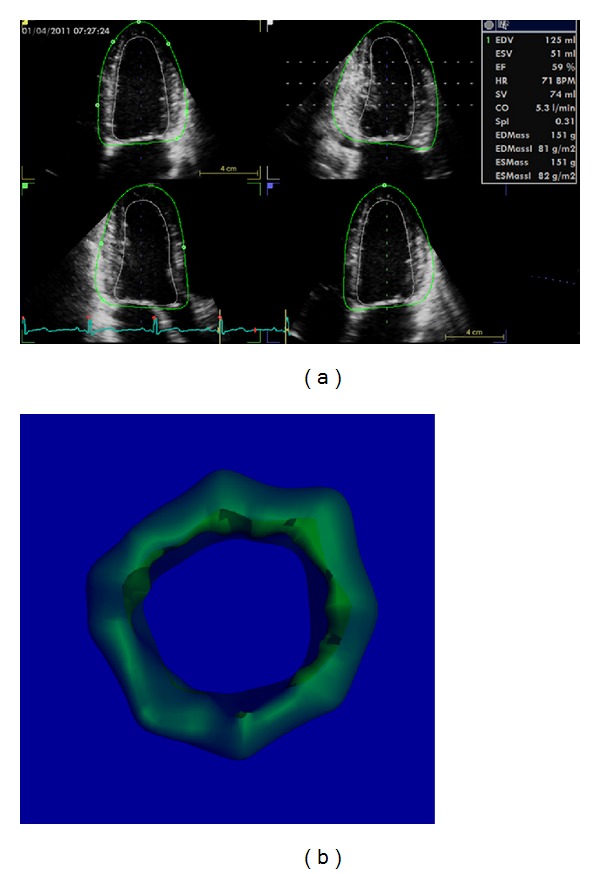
Left ventricular mass measurement using three-dimensional echocardiography. Using automated or semiautomated endocardial and epicardial boundary detection endocardial and epicardial volumes are measured (a). By subtracting the left ventricular cavity volume from the epicardial volume, the volume of myocardium is obtained (b). Left ventricular mass is calculated by multiplying myocardial volume by its specific gravity (1.05 g/cm^3^).
